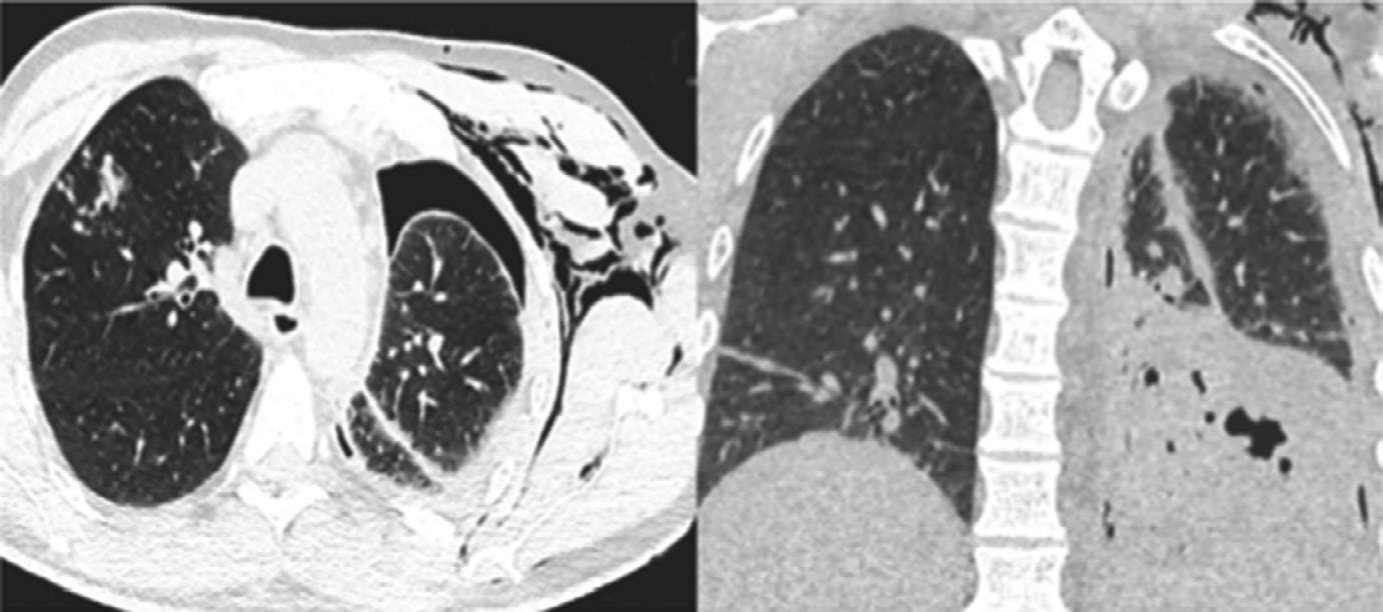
Atypical CT imaging features for COVID‐19. Unenhanced axial and coronal CT images showing tree‐in‐bud nodules with lobar consolidation of the left lower lobe and left side pneumothorax and subcutaneous emphysema
